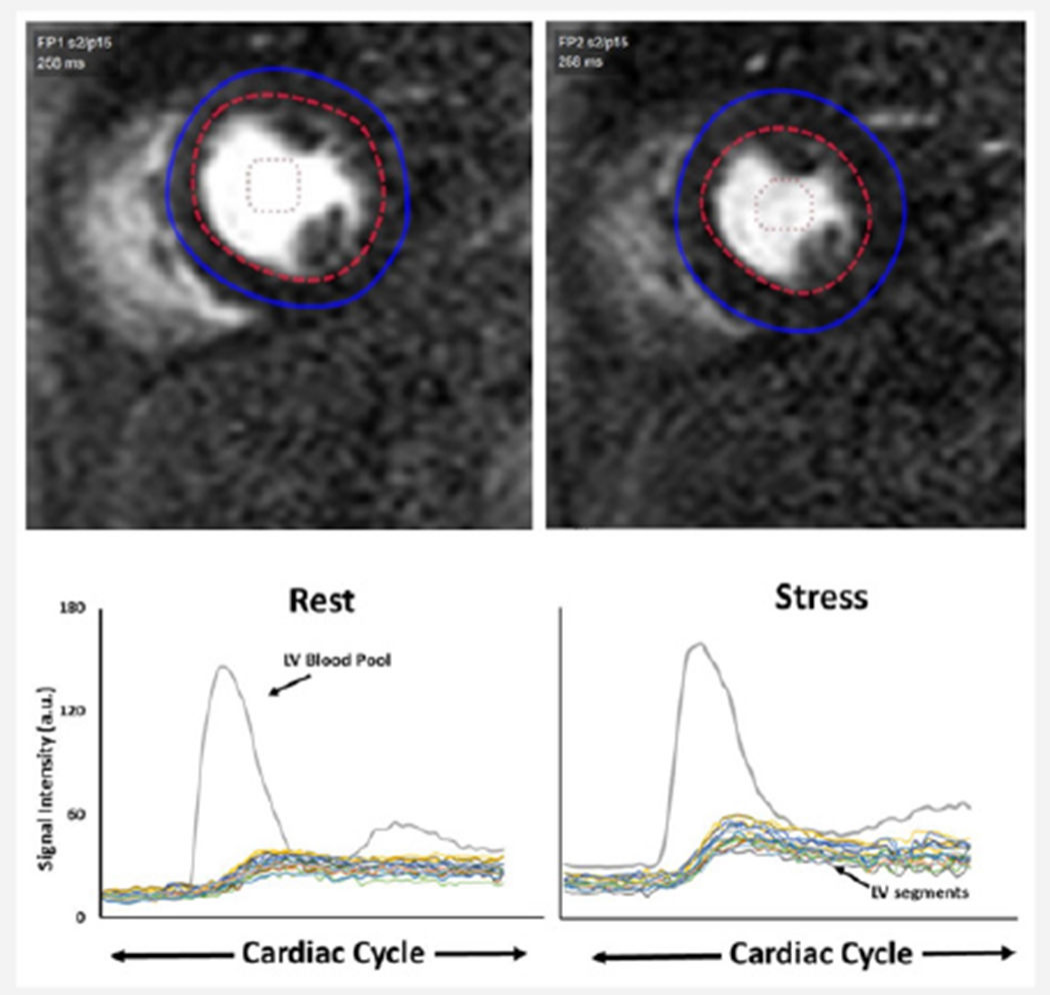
Cardiac Magnetic Resonance Imaging (CMRI) at rest and first pass myocardial perfusion images with corresponding intensity-over-time curves. Signal intensity for the mid-left ventricular cavity (green curve) and for the 6-segments myocardial first pass increment in signal.
